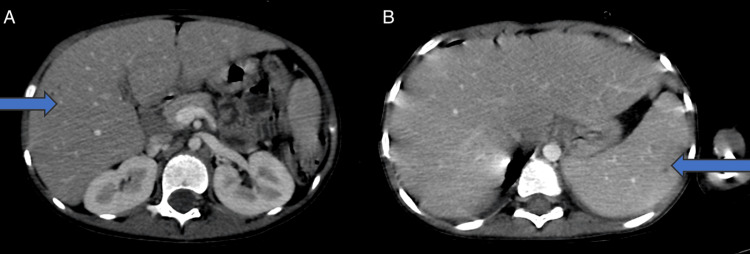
Axial views of an abdominal CT scan showing microabscesses in the liver (A) and spleen (B)
